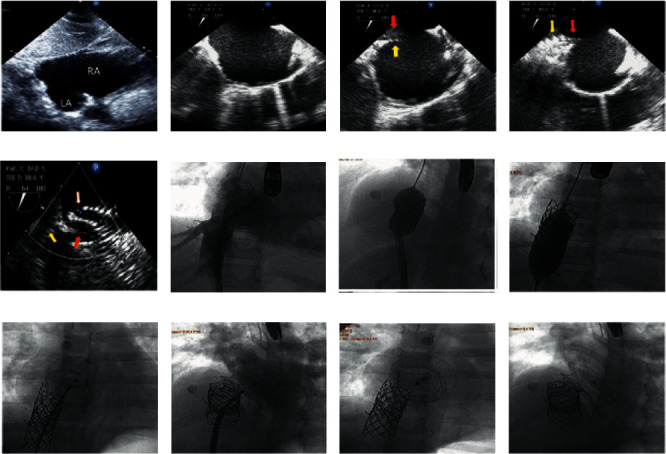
(a) Transthoracic echocardiogram, subcostal bicaval view demonstrates a large secundum ASD with an absent inferior rim. (b) TEE in long axis bicaval view shows large secundum ASD with absent inferior rim with right sided volume overload. (c, d) TEE in long axis view demonstrates the struts of the distal part of the inflated CP stent (yellow arrow) at the entrance of the IVC creating a new inferior rim (red arrow). (e) TEE imaging in a modified long axis view demonstrates struts of the inflated uncovered CP stent (yellow arrow) at the entrance of the IVC creating a new inferior rim (red arrow) which has been captured by two discs of the ASO (white arrow). (f) Cine angiogram of the IVC in frontal view demonstrates the uncovered CP stent mounted over the *Z*-med balloon size (25 × 40 mm) at the entrance of the IVC under the guidance of the TEE for proper position of the stent. (g) Cine imaging of the heart in four chamber view demonstrates the inflation of the uncovered CP stent, 8 zig, 45 mm, using *Z*-med balloon size (25 × 40 mm) at the entrance of the IVC into the RA under the guidance of the TEE. (h) Cine imaging of the heart in frontal view demonstrates the inflation of the second CP-stent 8 zig, 39 mm, overlapping with previous stent for proper fixation. (i) Cine imaging of the heart in the frontal projection demonstrates the proper deployment of the Amplatzer septal occluder size 34 mm before release. (j) RA angiogram in four chamber view through cook sheath 14F demonstrates the deployed septal occluder which was well aligned with IAS. (k) Cine imaging of the heart in aneteroposterior projection demonstrates both the two overlapping uncovered CP stents in the IVC with the proper position of the atrial septal occluder. (l) Cine imaging of the heart in four chamber projection demonstrates two overlapping uncovered CP-stents and the device which is well aligned with IAS under the guidance of the TEE, the device is not anchored the CP stent at all.
